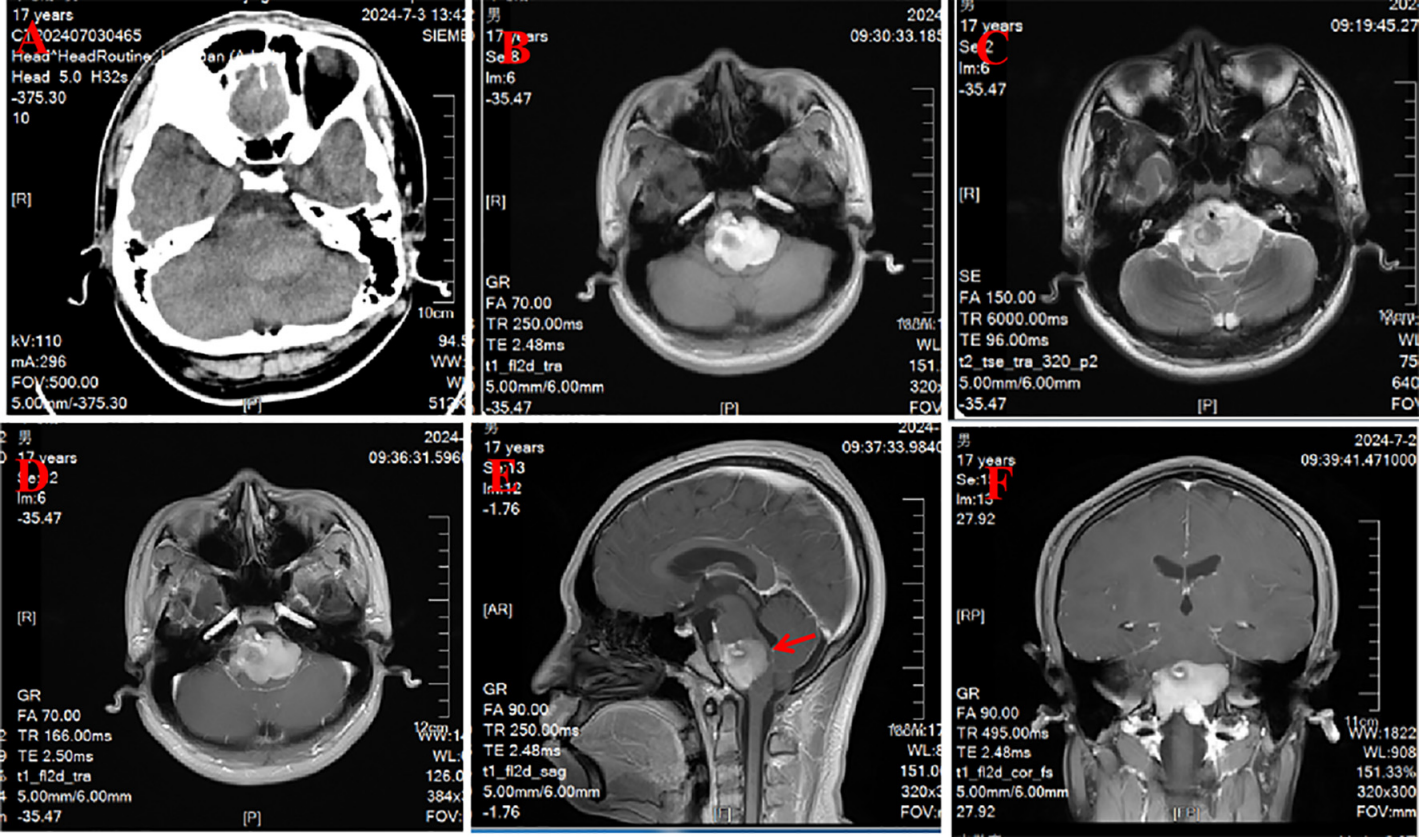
Preoperative CT and MRI results. (A) CT scanning showed a cauliflower-like hyperdense lesion located in the brainstem. (B, C) The lesion was mostly hyperintense on T1- and T2-weighted scanning. (D–F) Enhanced scanning showed that the lesion was a prominent gadolinium enhancement.
